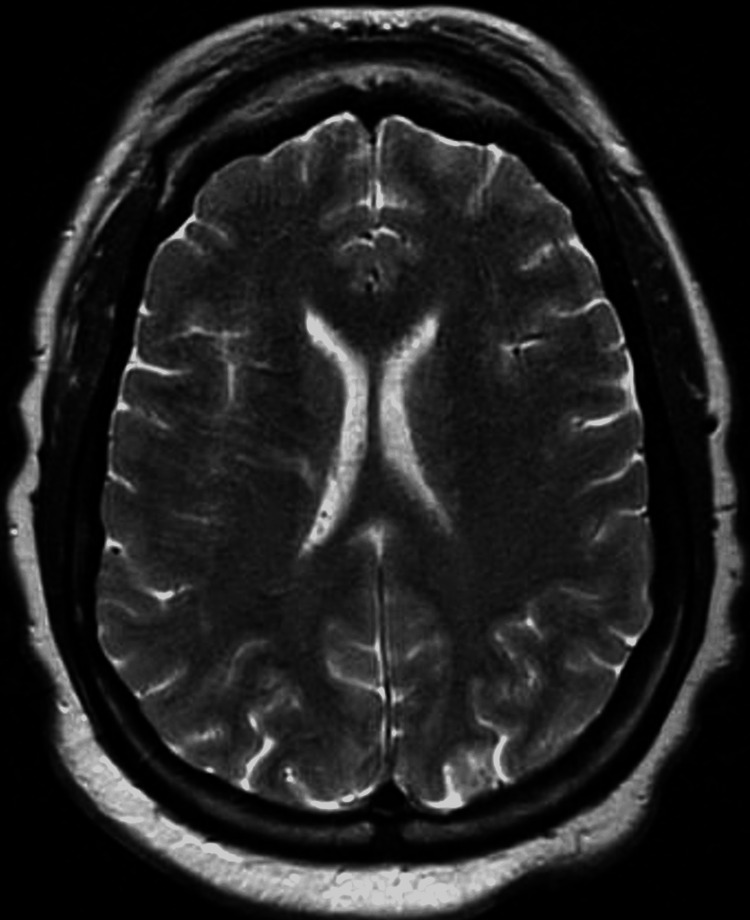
T2 magnetic resonance imaging brain showing grossly unremarkable intracranial findings.
